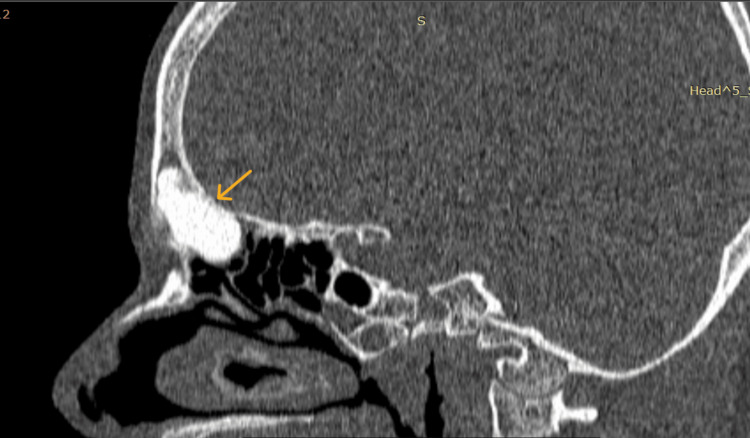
Sagittal section of CT PNS showing a well-defined, hyperdense bony density mass in the frontoethmoid region with broad-based attachment to the frontal bone and ethmoid roof with adjacent frontal bone thinning and endosteal scalloping. There was no evidence of the mass extending into the intracranial region, and the posterior wall of the frontal sinus appeared intact. CT, Computed tomography; PNS, Paranasal sinus.
